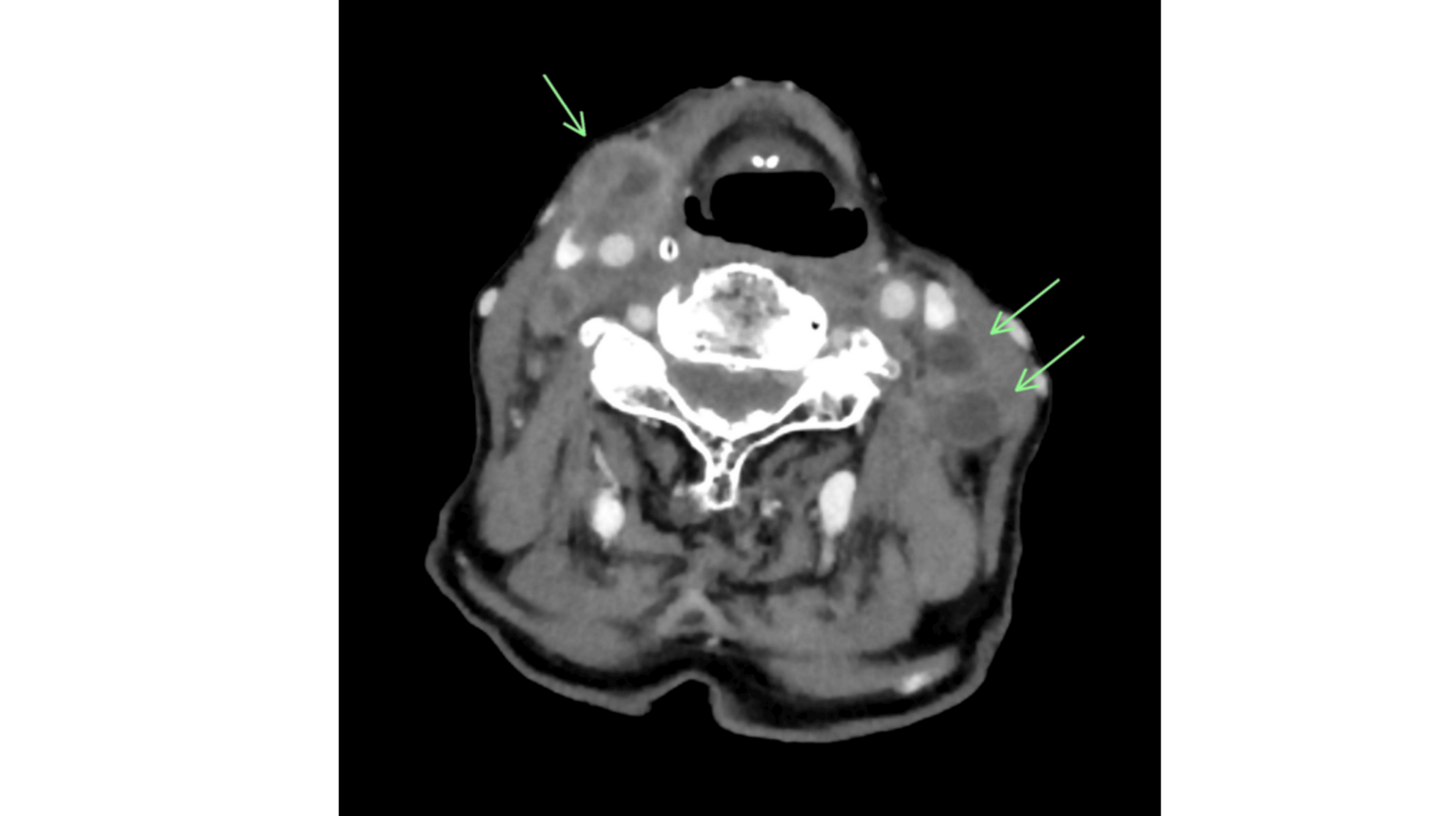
Computed tomography findings post-stereotactic radiotherapy. A complete response was achieved, with resolution of the submental lymph node metastasis; however, bilateral cervical lymph node enlargement and metastases were observed.
